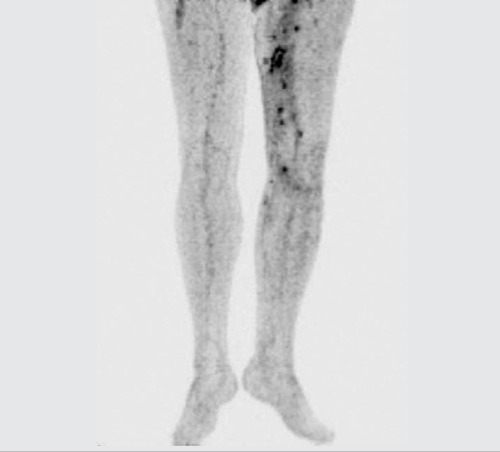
MIP image of lower extremity showed multiple foci ofFDG uptake (SUV_max_:6.6) on the left thigh and the medial side ofthe knee within the subcutaneous tissue
